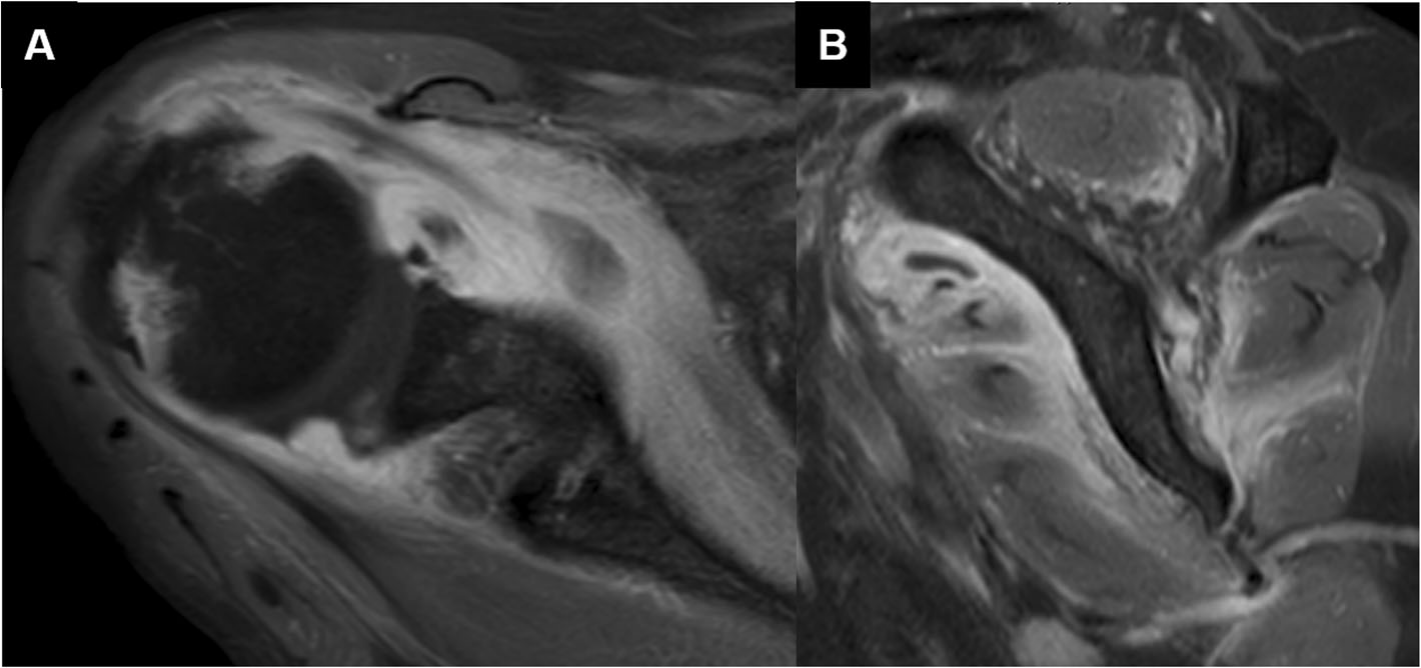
Gadolinium-enhanced fat-suppressed T1-weighted MRI oblique axial (a) and oblique sagittal (b) images revealed widespread contrast-enhanced inflammation and abscess formation within the right subscapularis muscle and glenohumeral joint space
